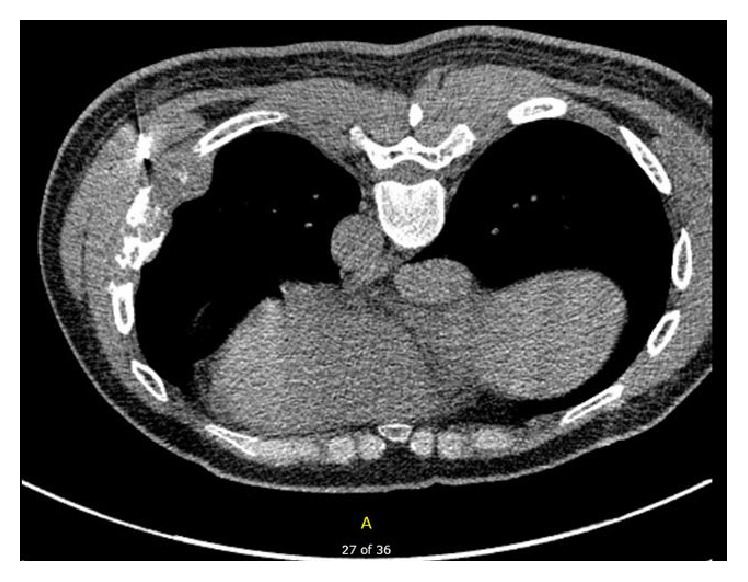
Axial CT chest without contrast, soft tissue views demonstrating the patient in prone position with CT-guided biopsy needle on satisfactory trajectory to the bony and soft tissues lesion.
